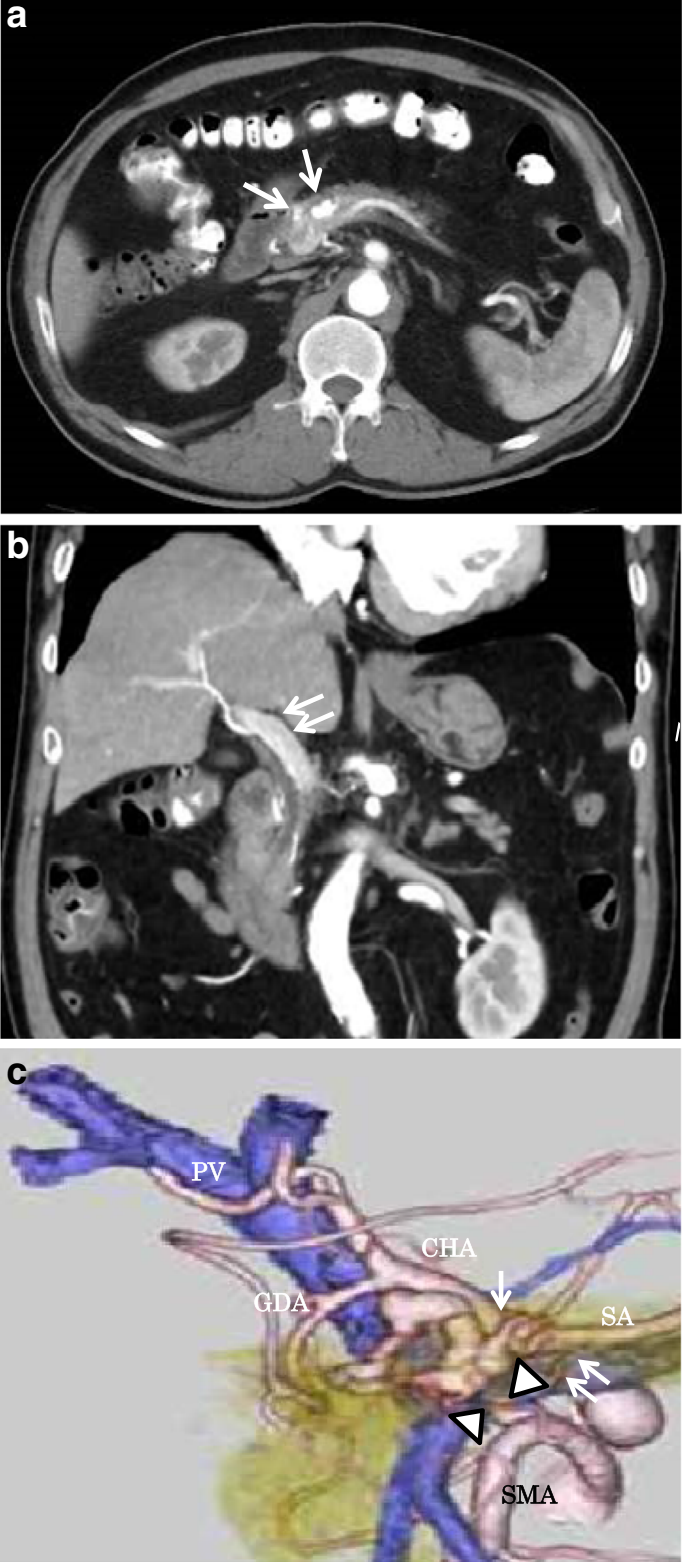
Computed tomography scan showed irregular dilatation with meandering in the pancreatic head (*single arrow*; a) and the early appearance of the portal vein at the arterial phase (*double arrows*; b). Three-dimensional imaging showed feeders from the superior mesenteric artery (SMA) (*arrow heads*), celiac artery (*single arrow*), and splenic artery (*double arrows*) (c). *PV* portal vein, *CHA* common hepatic artery, *GDA* gastroduodenal artery, *SA* splenic artery
